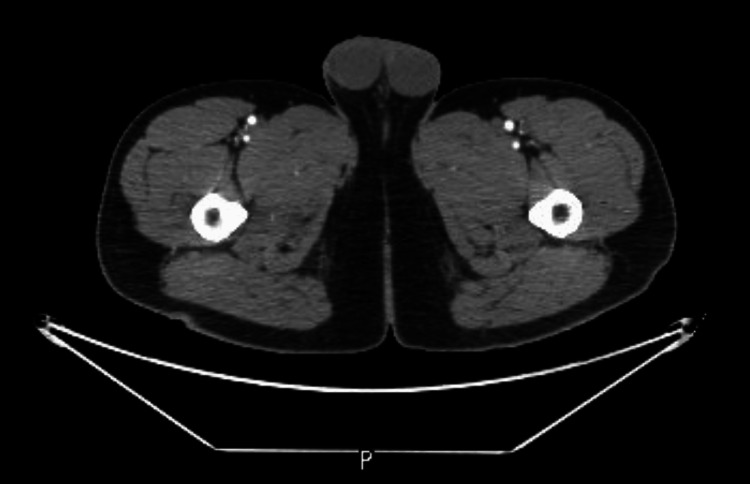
Pelvic computed tomography (CT) of the scrotum demonstrating normal testicular morphology. Pelvic CT scan imaging of the scrotum demonstrates bilaterally symmetric testes with preserved size and homogeneous parenchymal attenuation without evidence of focal lesions or structural abnormalities. This image was included to emphasize that normal-appearing testes do not exclude clinically relevant causes of azoospermia, including obstructive or congenital etiologies such as congenital bilateral absence of the vas deferens. Therefore, further diagnostic evaluation remains warranted despite preserved testicular morphology. CT: computed tomography.
